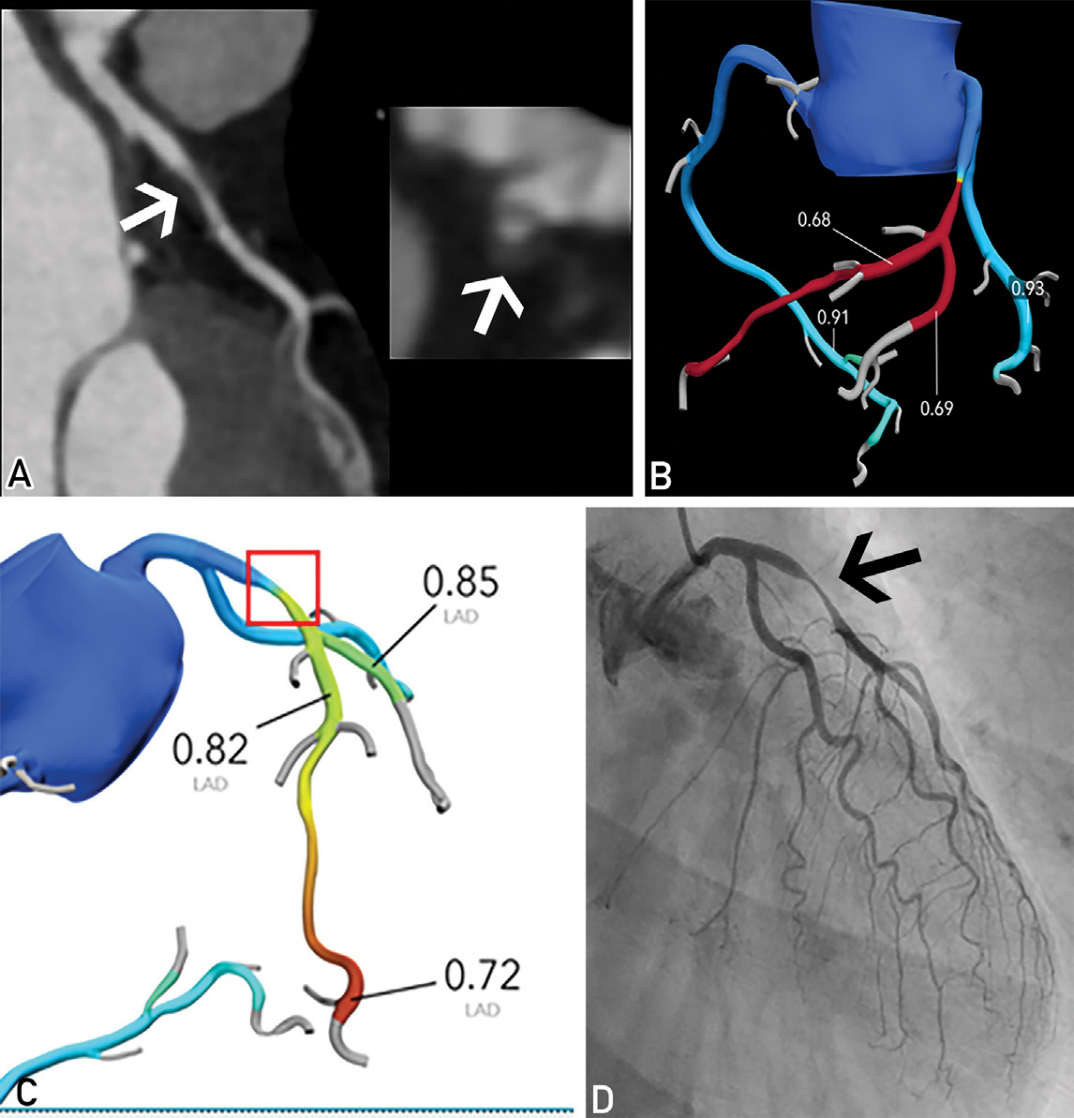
Segmentation error causing abnormal CT-FFR results.^1^ A, Curved multiplanar reconstruction and axial image show severe stenosis of mid-LAD. B, Abnormal CT-FFR value is noted in LAD distal to the lesion. The modeling of the narrowed LAD segment is tighter than what is observed in the CTCA image. C, CT-FFR image after correction in modeling the LAD segment. The CT-FFR value distal to the lesion is 0.82. D, ICA image shows a severe stenosis in mid-LAD. The invasive FFR value was 0.8, complying with the CT-FFR results. Pictures are adapted from Rajiah et al.^1^ CT-FFR, computer tomography–derived fractional flow reserve; ICA, invasive coronary angiogram; LAD, left anterior descending artery.
